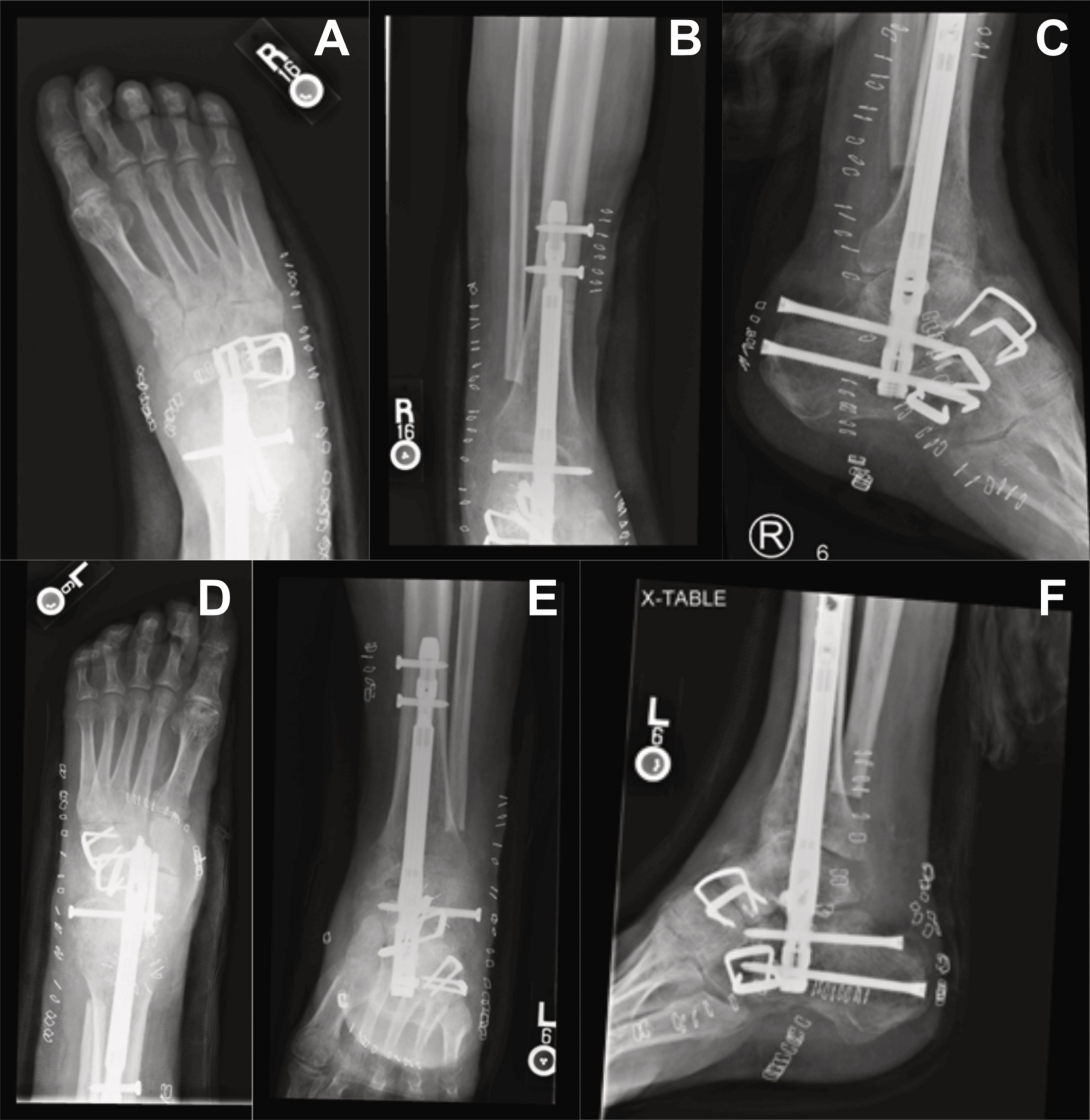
Postoperative radiographic views following bilateral pantalar arthrodesis A: Right foot anteroposterior view, B: right ankle anteroposterior view, C: right ankle lateral view, D: left foot anteroposterior view, E: left ankle anteroposterior view, F: left ankle lateral view
